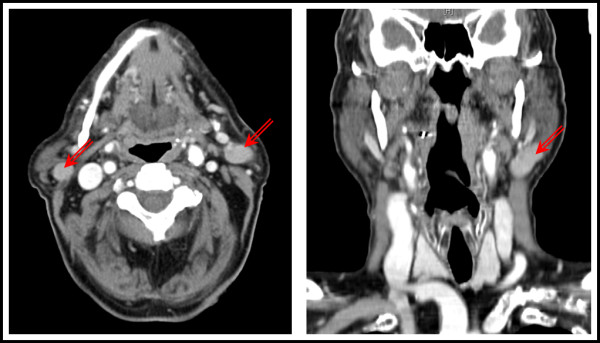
Contrast-enhanced CT-scans showed a lesion next to the angle of the mandible on both sides (red arrows). The ovoid lesion showed a defined border with an intense uptake of the contrast medium.
